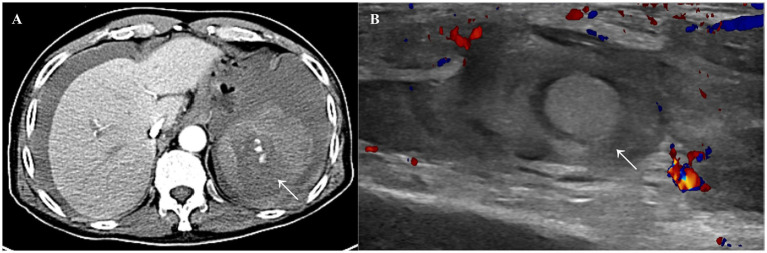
(A) Contrast-enhanced abdominal CT revealed splenic rupture complicated by hemoperitoneum. (B) Ultrasound examination suggested a hypoechoic mass in the left forearm, with a size of approximately 2.6 × 2.2 × 4.6 cm, which was considered to be a hematoma.
